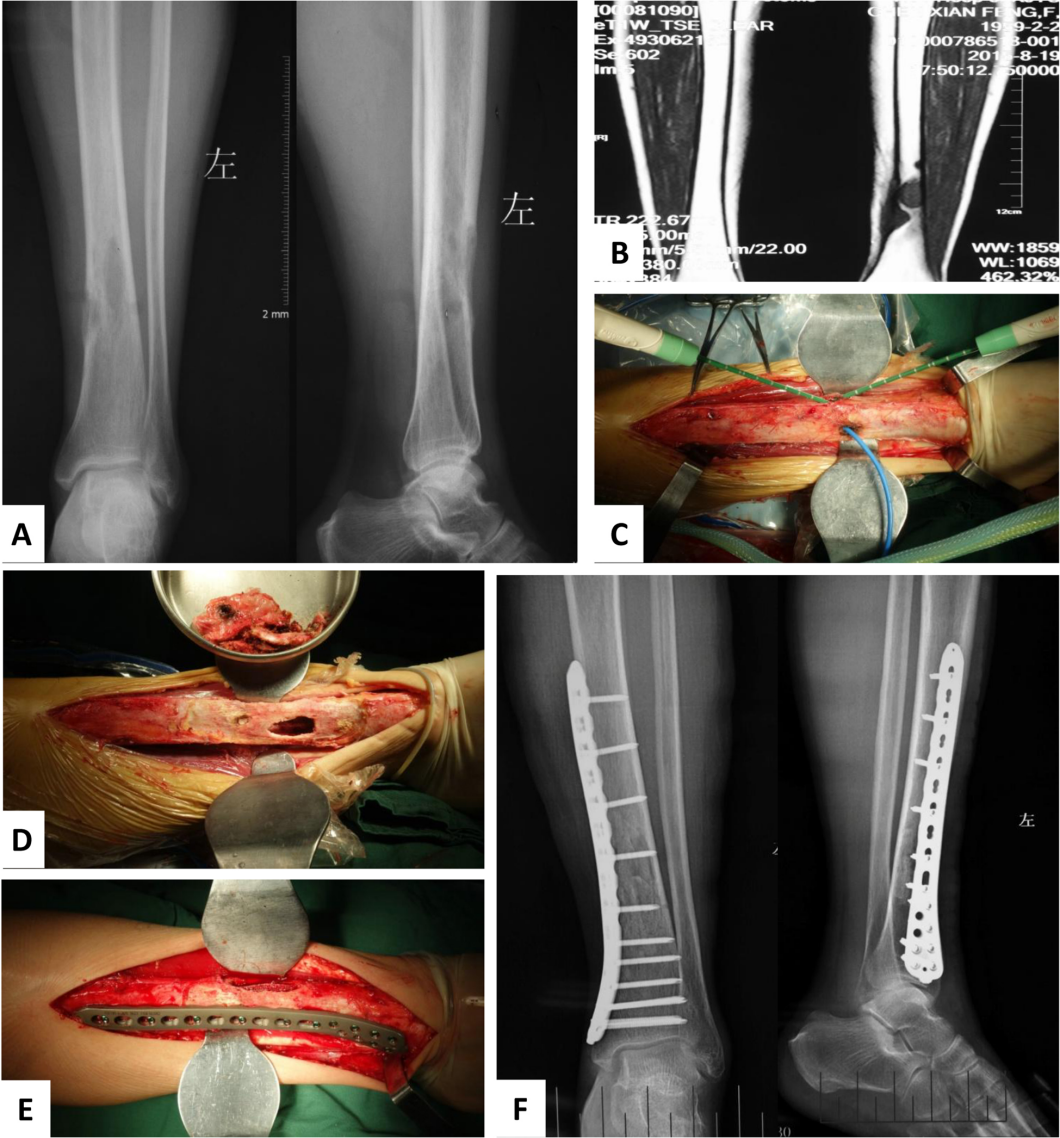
Typical procedure for malignant bone tumors at tibial diaphysis. a Image data of A-P and lateral X ray films showed a metastatic neoplasm from primary thyroid carcinoma to distal tibial diaphysis before surgery. The vague boundary of the bone defect demonstrated that the lesion might be malignant. b Preoperative MRI imaging film demonstrated the accurate location and involved range of the metastatic lesion. c The microwave generator and antenna were inserted into the tumor bulk and began to deliver electromagnetic energy into the tumor bone. d Tumor bone inside the tibial diaphysis was removed and bone graft cavity was prepared. e The allograft bone fragments were used to fill the bone defect and a prophylactic fixation with was conducted using molding steel plate. f Postoperative A-P X and lateral ray films demonstrated the tumor removal and tibia rebuilding
